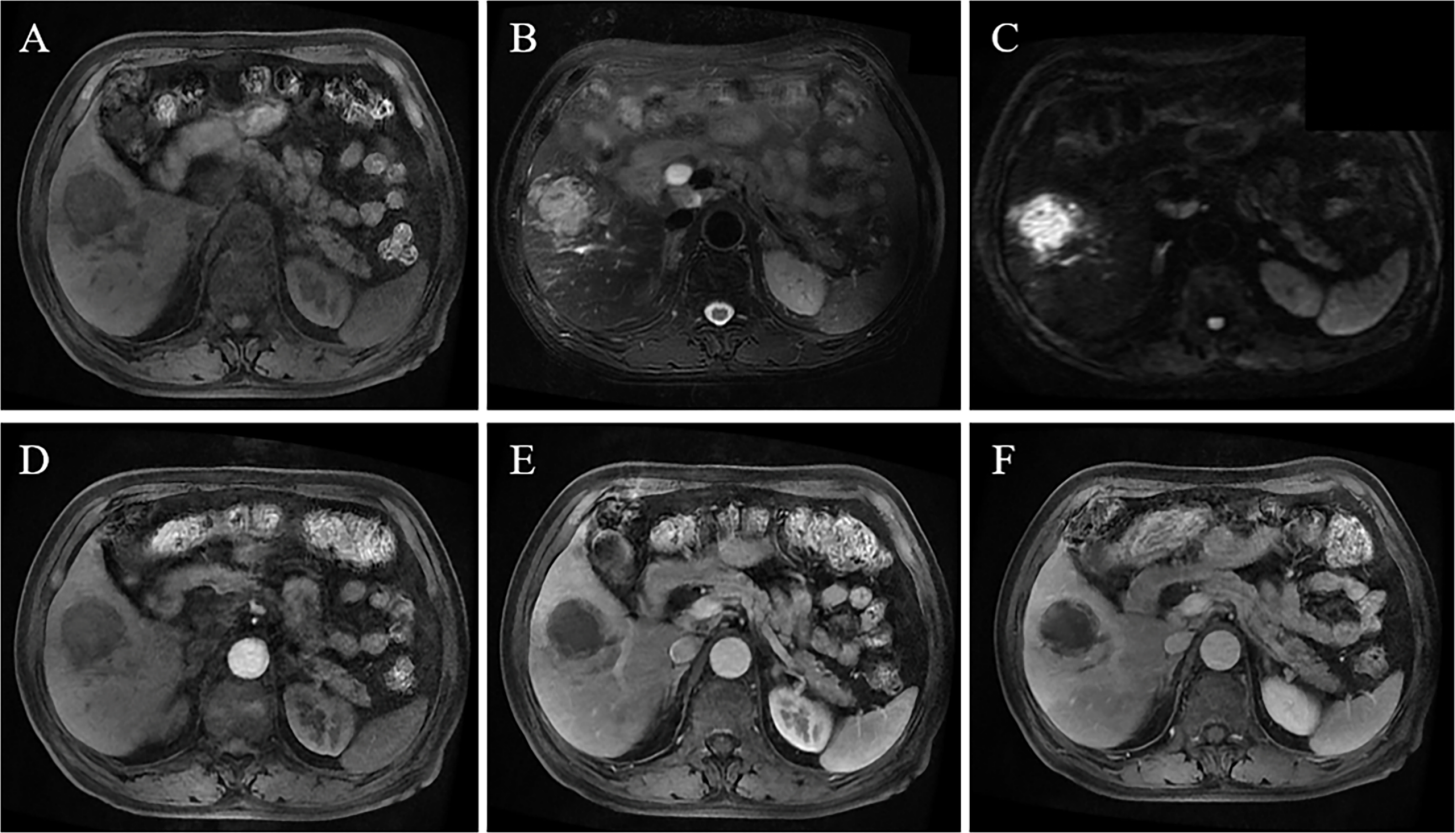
A 75-year-old male patient had fever symptoms. MRI reveals a 4.4-cm mass in the right lobe, with hypointensity on T1WI (A), slightly hyperintense signal on T2WI (B), and high signal on DWI (C); contrast-enhanced MRI shows low enhancement in the arterial phase (D), portal vein phase and delayed phase (E, F), indicating a poor blood supply.
